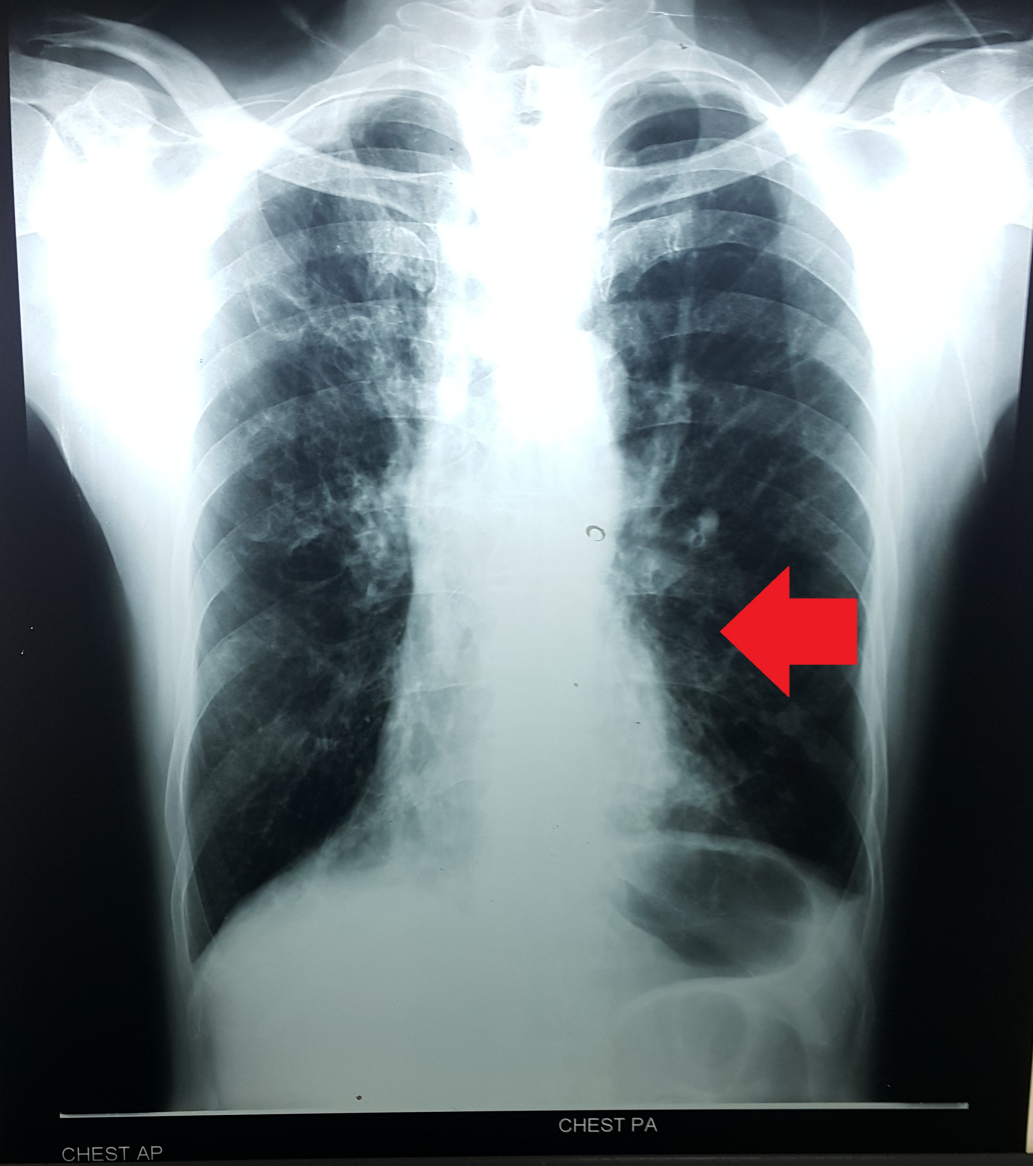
Chest x-ray showing mild cardiomegaly with pulmonary congestion and dextrocardia
